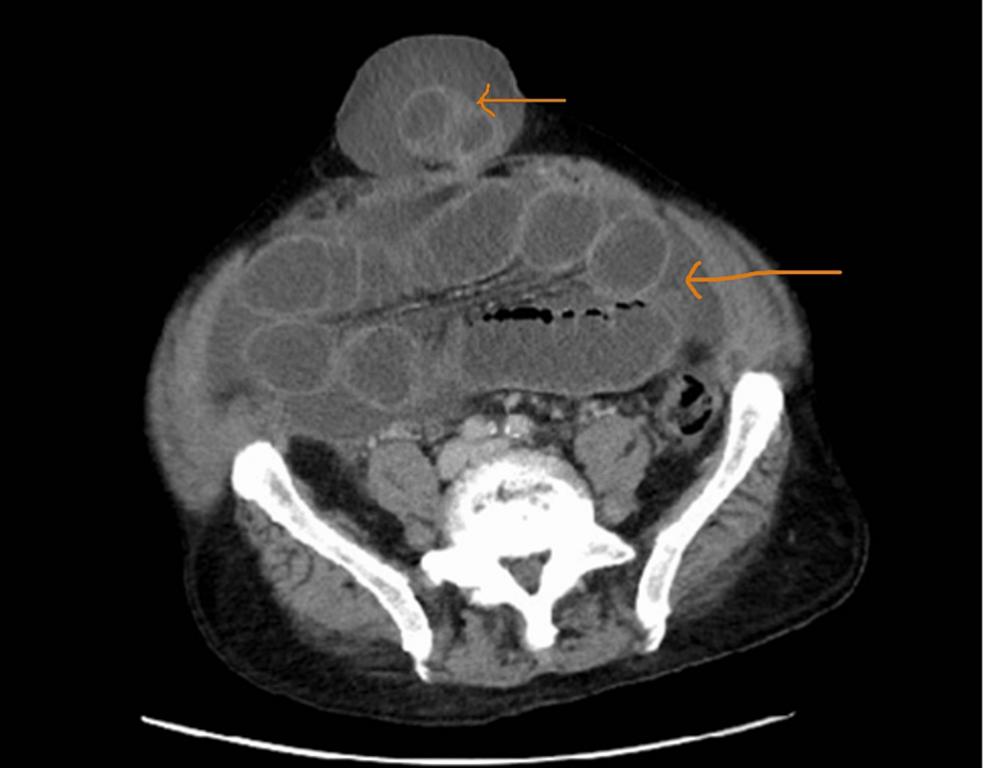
CT scan of the abdomen without contrast on admission prior to abdominal paracentesis CT scan of the abdomen without contrast on admission prior to abdominal paracentesis, showing abdominal ascites (orange arrow), and umbilical hernia with no contents (red arrow).
